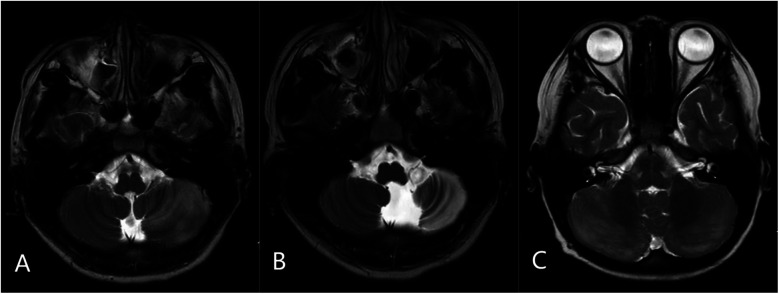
Representative MRI findings in acute cerebellitis (AC): (A) axial T2-weighted image showing diffuse hyperintensity and swelling in the left cerebellar hemisphere. (B) Follow-up axial T2-weighted image at 2 years in the same patient demonstrating left cerebellar atrophy. (C) Axial T2-weighted image from another patient showing bilateral cerebellar diffuse hyperintensity with swelling.
